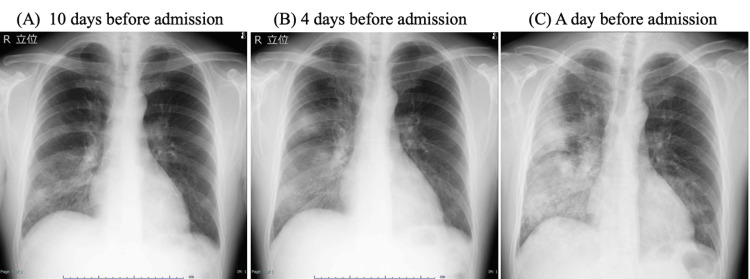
Serial chest X-ray imaging of the patient. (A) 10 days, (B) four days, and (C) one day before admission, showing progressive pulmonary infiltrates initially in the right lower lung, extending to the upper and left lung fields.
